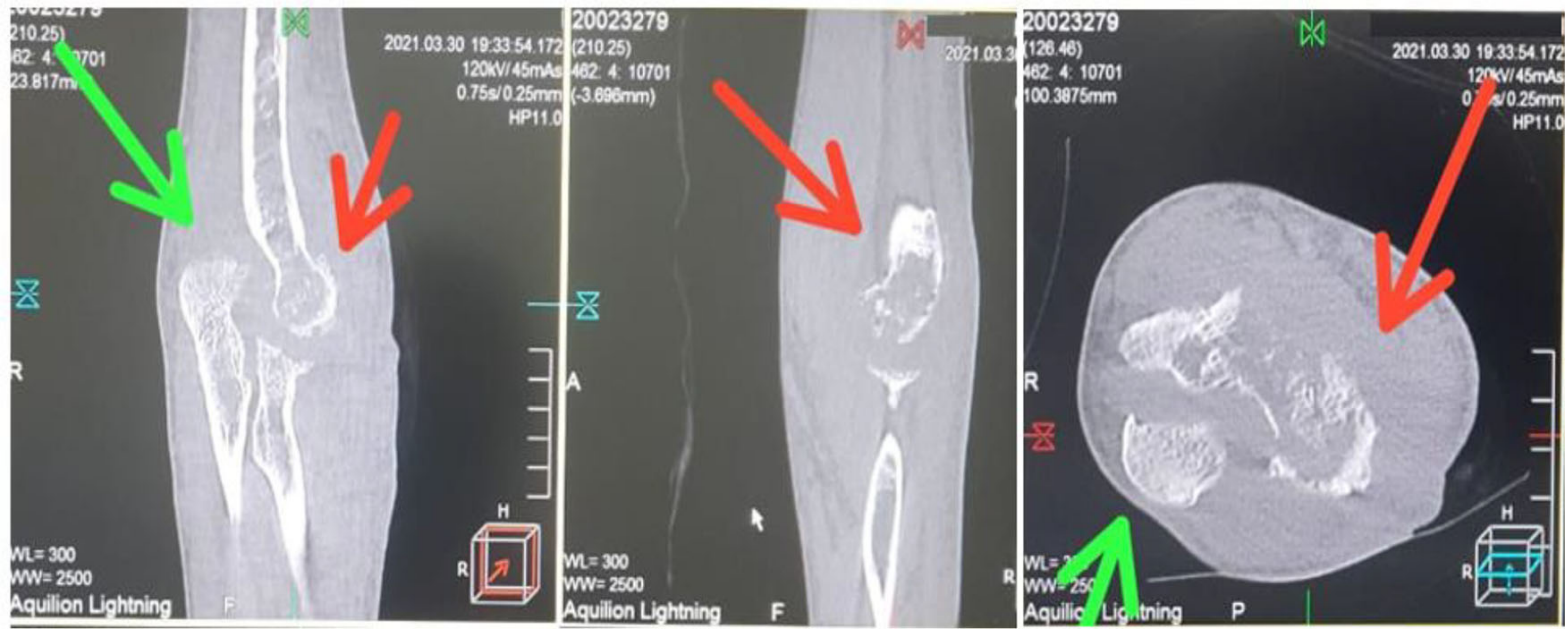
Computed tomography scan shows destruction of the lateral epicondylus of the humeral bone and the processus olecranon of ulna bones (red arrows = humerus bones, green arrows = ulna bones). Picture was edited with photoshop CS4 version 11.0 to remove specific details of dates of patient care and patient’s identity.
